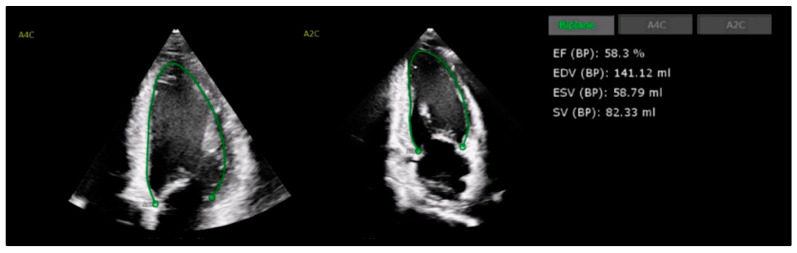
Example of AI model for automated measurement of left ventricular ejection fraction (LVEF), reported on apical 4-chamber (A4C) and apical 2-chamber (A2C) views. Model utilizes endocardial contour auto-generation to trace end-diastolic LV cavity volume (EDV), subsequently reporting LVEF by Simpson’s method.
